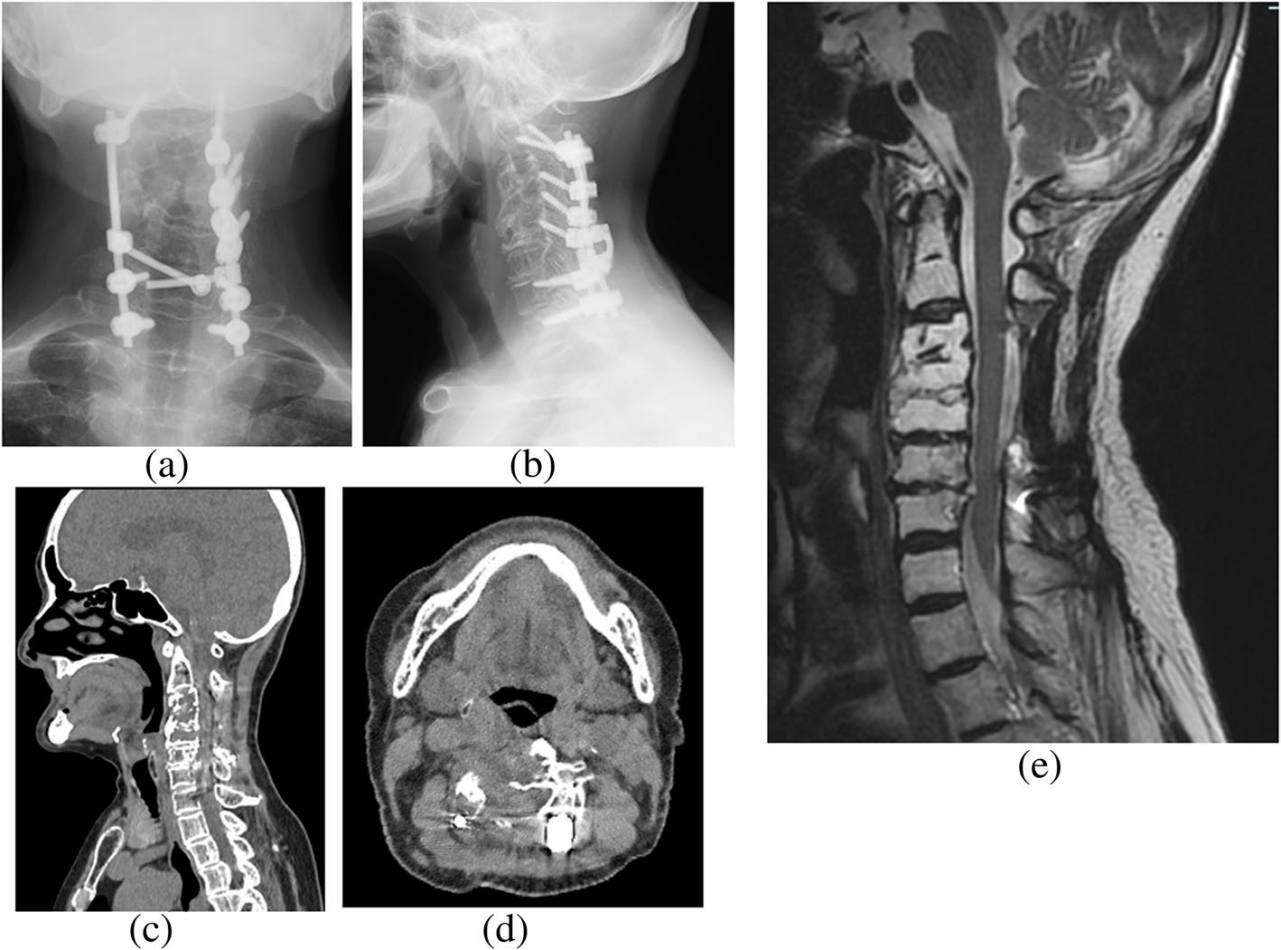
Radiographs, cervical CT, and MRI T2-weighted images at 18 months after surgery. There were no signs of recurrence and no significant changes in the osteolytic lesions on CT
